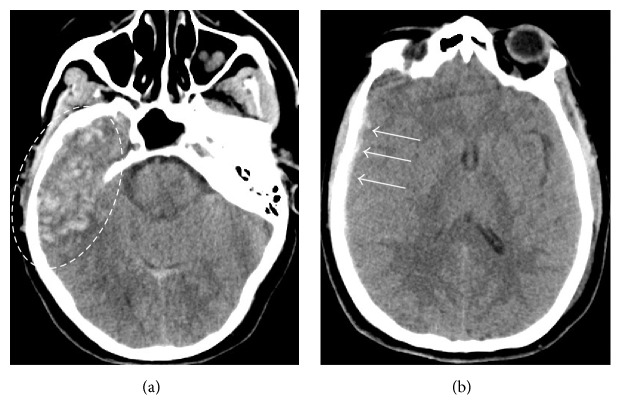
Computed tomography (CT) of the head. CT head showed a right temporal contusion with mixed density and surrounding vasogenic edema (dashed circle; (a)). She also had a small right frontal subdural hemorrhage (arrows; (b)).
